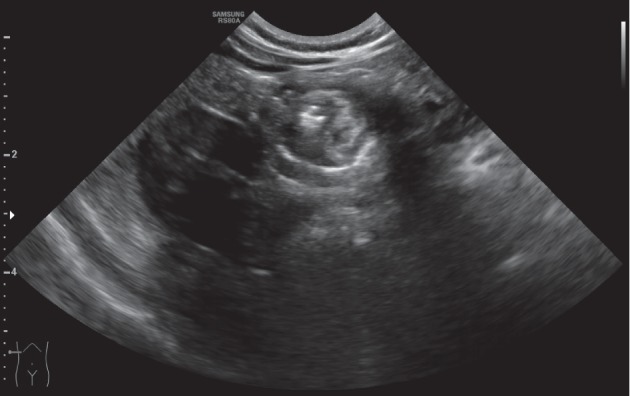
The classical target sign seen at ultrasound which prompted the hydrostatic enema.
